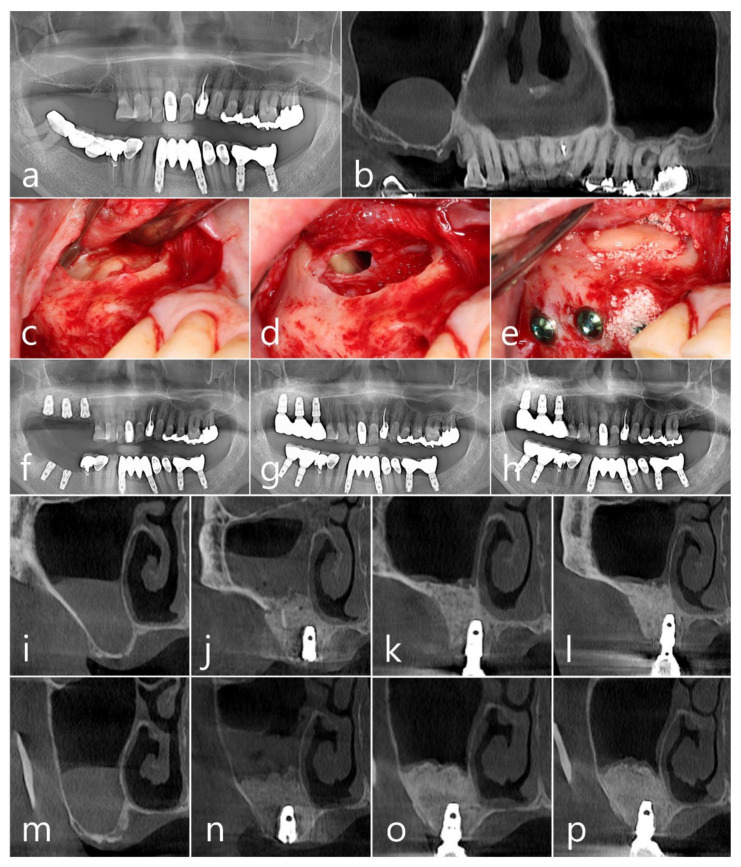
Case 2. Preoperative radiographs. A dome-shaped antral pseudocyst in the right maxillary sinus is shown in the panoramic radiograph (a) and CBCT (b). The “one-bony-window” technique showing lateral sinus window opening (c), intentional perforation at the upper part of the Schneiderian membrane and removal of antral pseudocyst (d), and sinus floor membrane elevation following bone grafting with implant placements, with repositioning of bony window (e). Panoramic view taken immediately after sinus floor augmentation and implant placement (f), after prosthetic delivery (g), and after 1 year of loading (h). Coronal CBCT images of the right maxillary first molar site before surgery (i), immediately after surgery (j), after prosthetic delivery (k), and after 1 year of loading (l). Coronal CBCT images of the right maxillary second molar site in the corresponding periods are described above (m–p).
